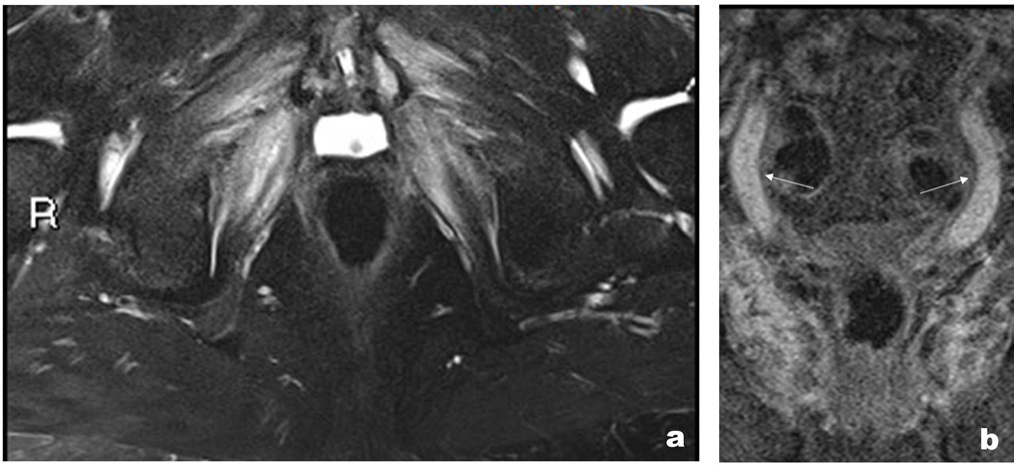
(a) MRI STIR axial sequence showing multiple fluid intensities involving the adductor muscles and the pubic symphysis in keeping with secondary osteomyelitis and myositis. (b) *T*
